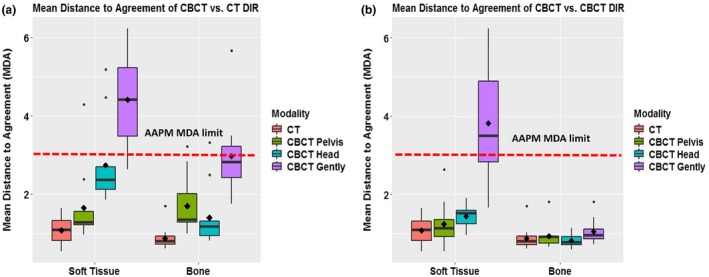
Accuracy of deformable image registration by mean distance to agreement. (a) Cross‐modality image registration from CBCT vs CT (also showed CT vs CT as a reference). (b) Same‐modality image registration from CBCT vs. CBCT (also showed CT vs CT as a reference). CBCT, cone‐beam computed tomography.
